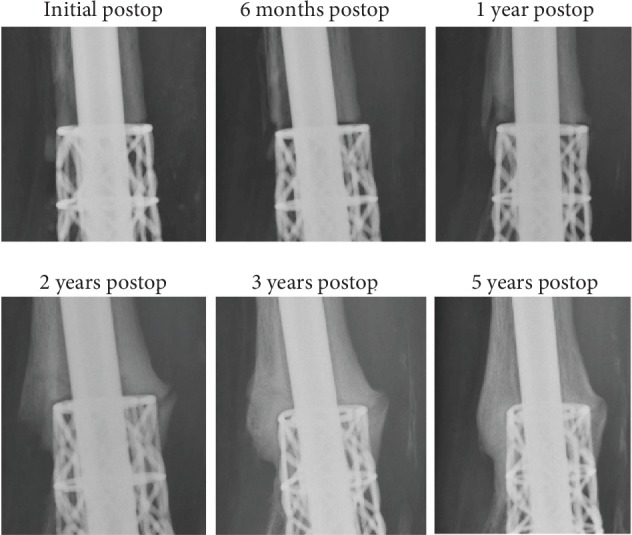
Serial radiographs demonstrating increased bone deposition at the interface of the distal tibia diaphysis and the proximal extent of the cage.
